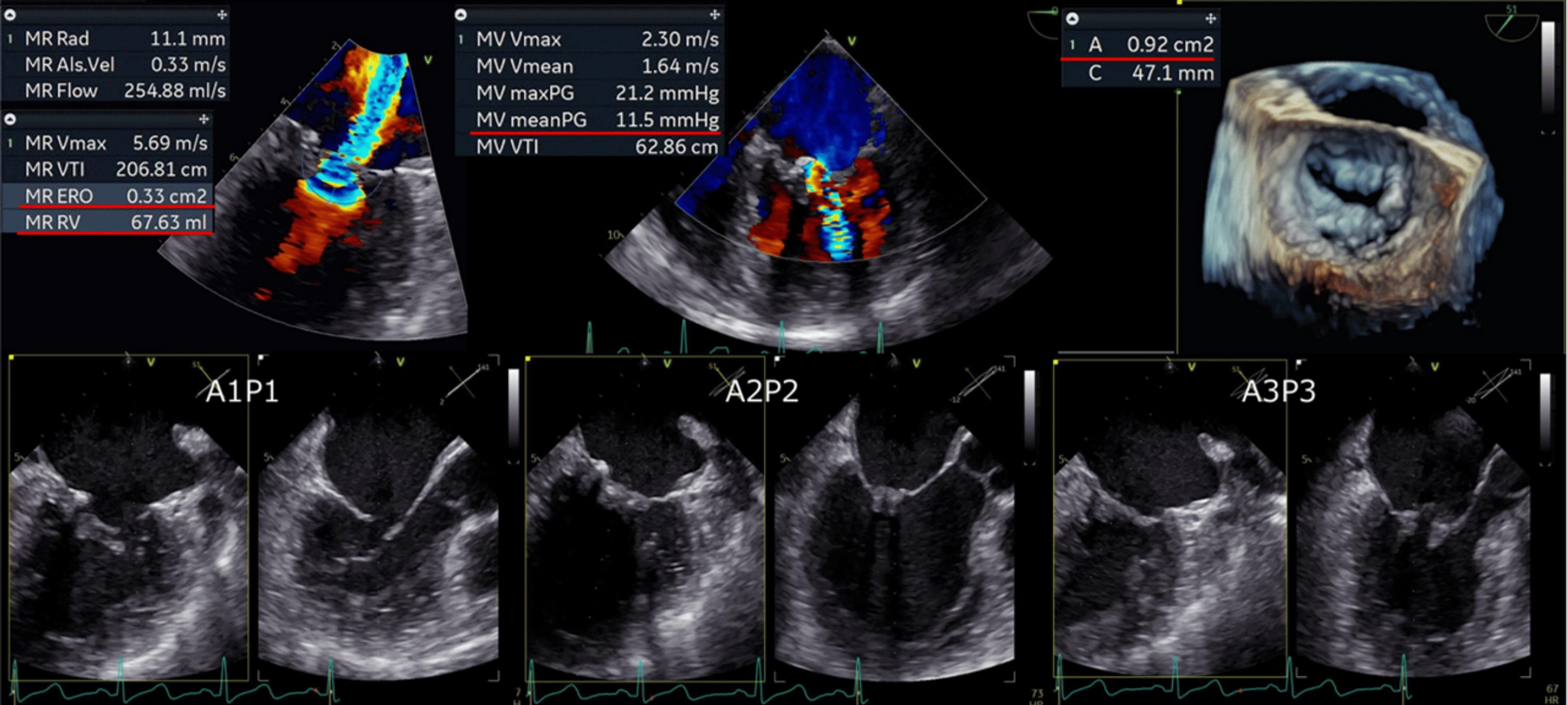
Transesophageal echocardiography data during the occurrence of DAH DAH: Diffuse alveolar hemorrhage
